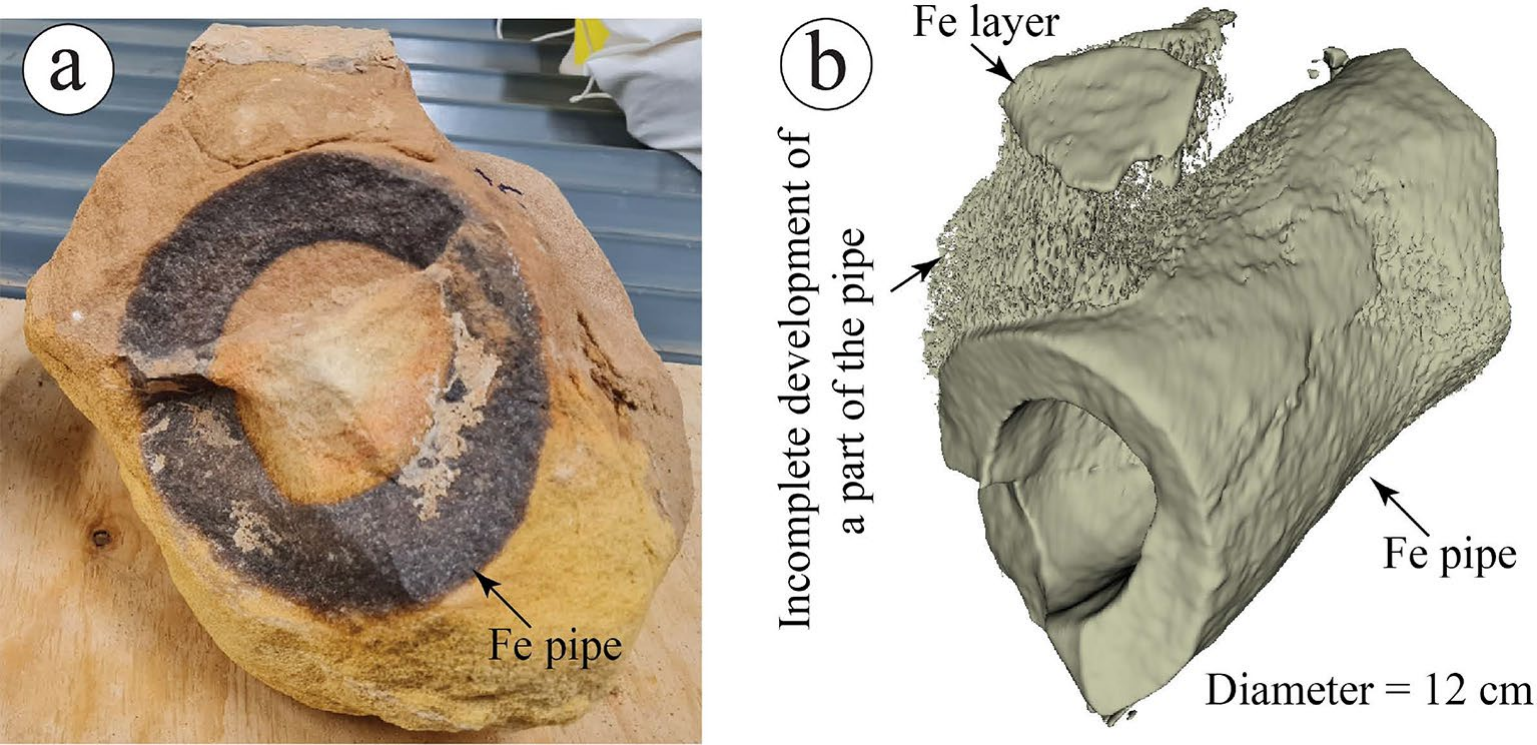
A three-dimensional view of a ferruginous (Fe) pipe (a) that can be visualized with the aid of CT scan (b, Supplementary Data).
